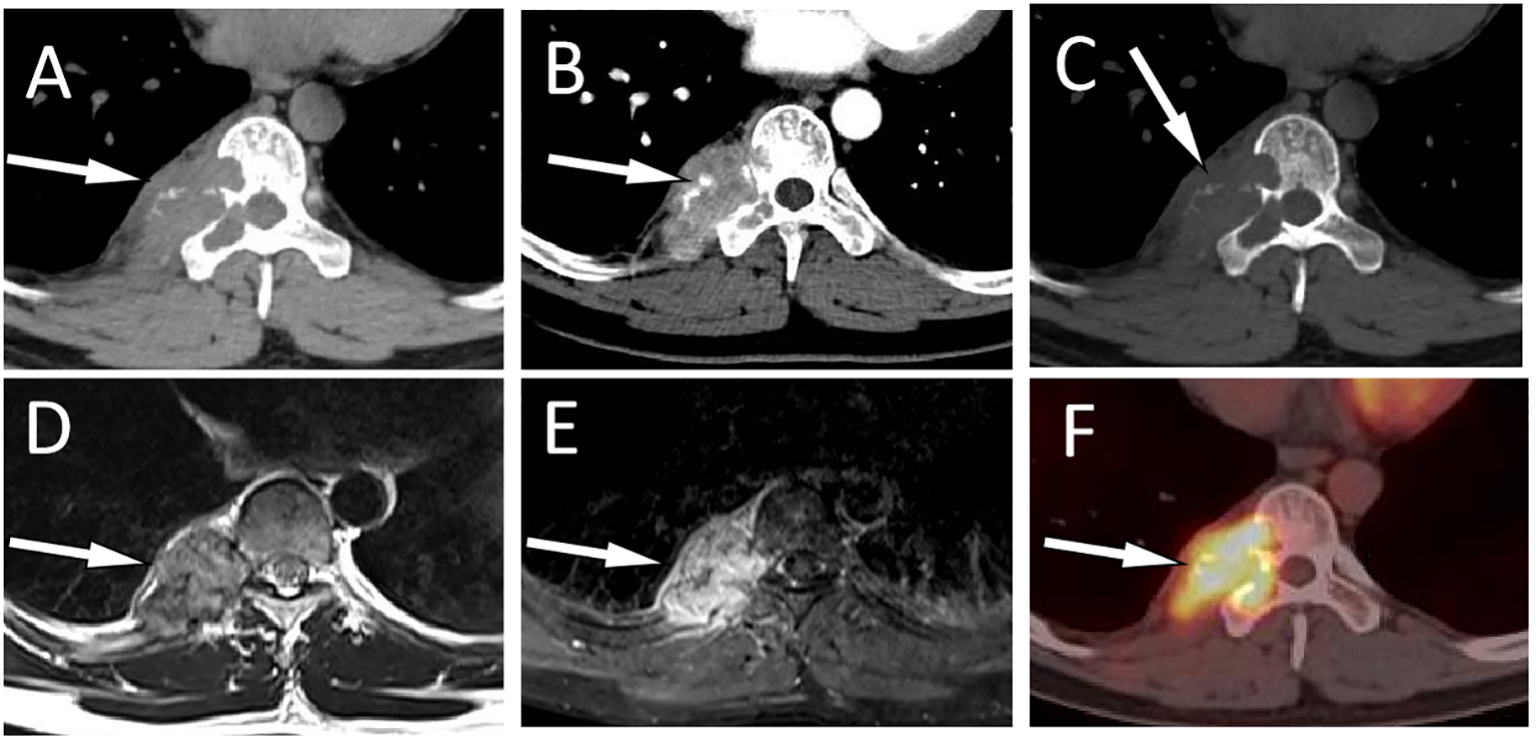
A 31-year-old man with EH (case 2): Axial CT soft tissue window displays a soft tissue mass extending toward the vertebral body and spinal canal centered around the right appendix of T9 [(A), arrow]. Contrast-enhanced CT showed obvious enhancement of the mass, with blood supply vessel shadows visible inside [(B), arrow]. The CT bone window shows expansive osteolytic bone destruction in the T9 vertebral body and the right accessory, with residual bone trabeculae visible inside (arrow) and no sclerotic rim (C). T2WI sagittal imaging shows a slight hyperintensity of the lesion [(D), arrow]. Fat-suppression T2 sequence showing hyperintensity of the lesion [(E), arrow]. Axial PET/CT fusion images (F) showed moderate FDG uptake (arrow), with an SUVmax of 7.5.
